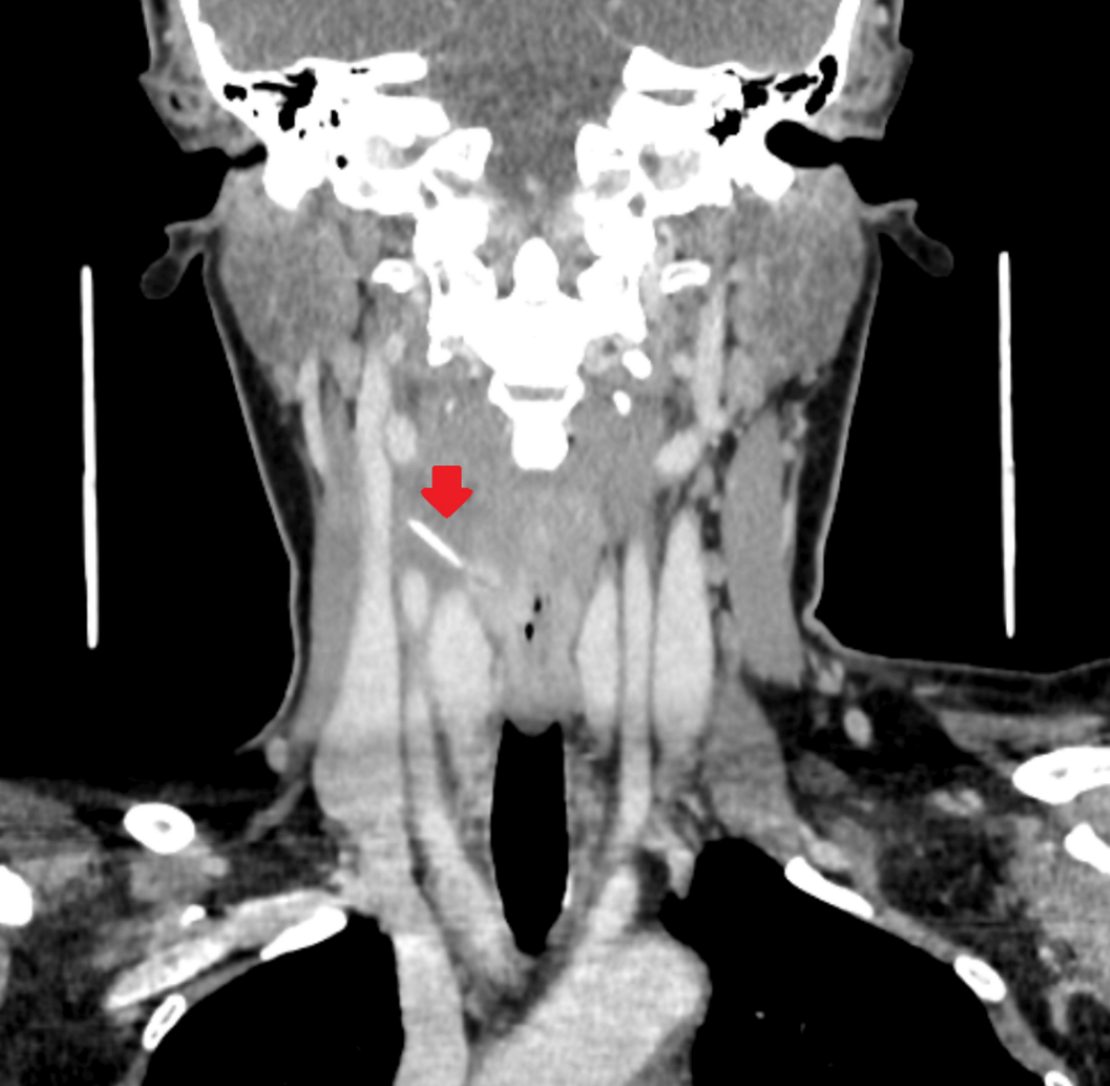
CT scan of the neck showing an impacted foreign body (red arrow) in the soft tissue of the neck. CT: computed tomography
